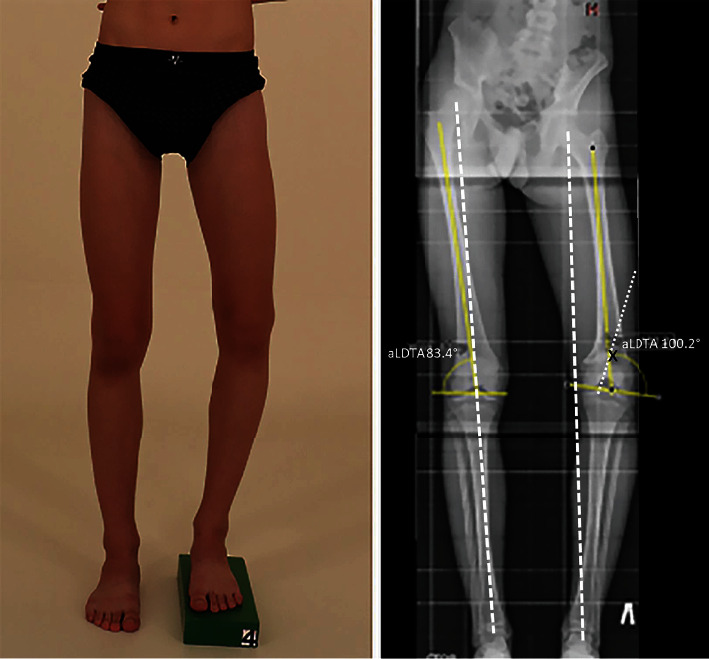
Preoperative photo and standing full-size radiograph of lower limbs: MAD (mechanical axis deviation) on the right side (0 mm) and on the left side (33 mm) of medial deviation; left side aLDFA (anatomical lateral distal femoral angle) 100.2°. Apex of the deformity (X) is situated at distal femoral metaphysis.
